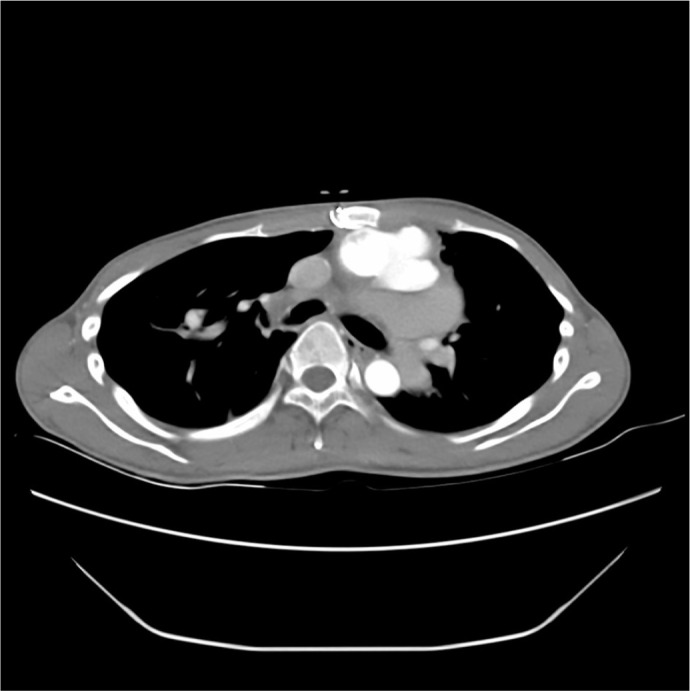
Preoperative computed tomography angiography tridimensional image
